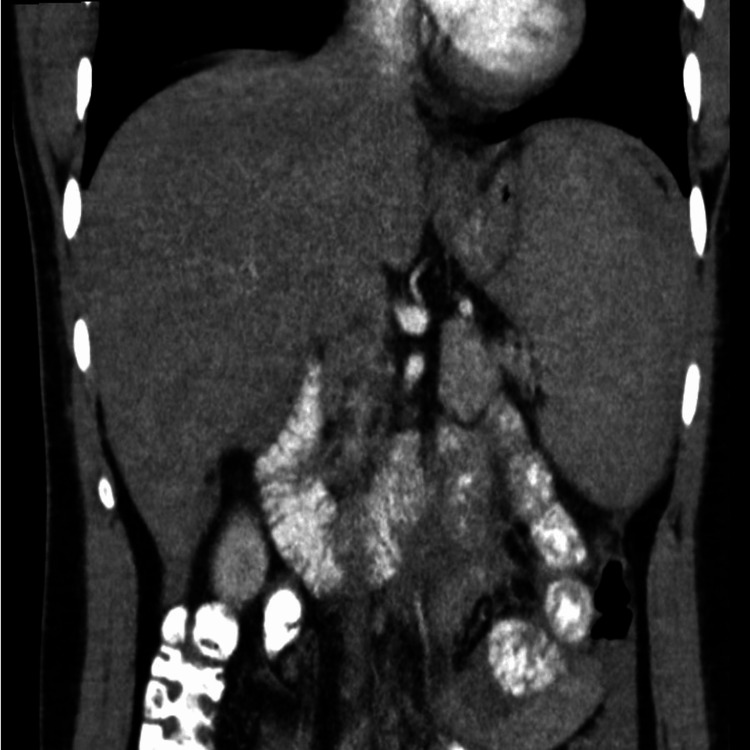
CT scan of abdomen with intravenous contrast in the sagittal plane
